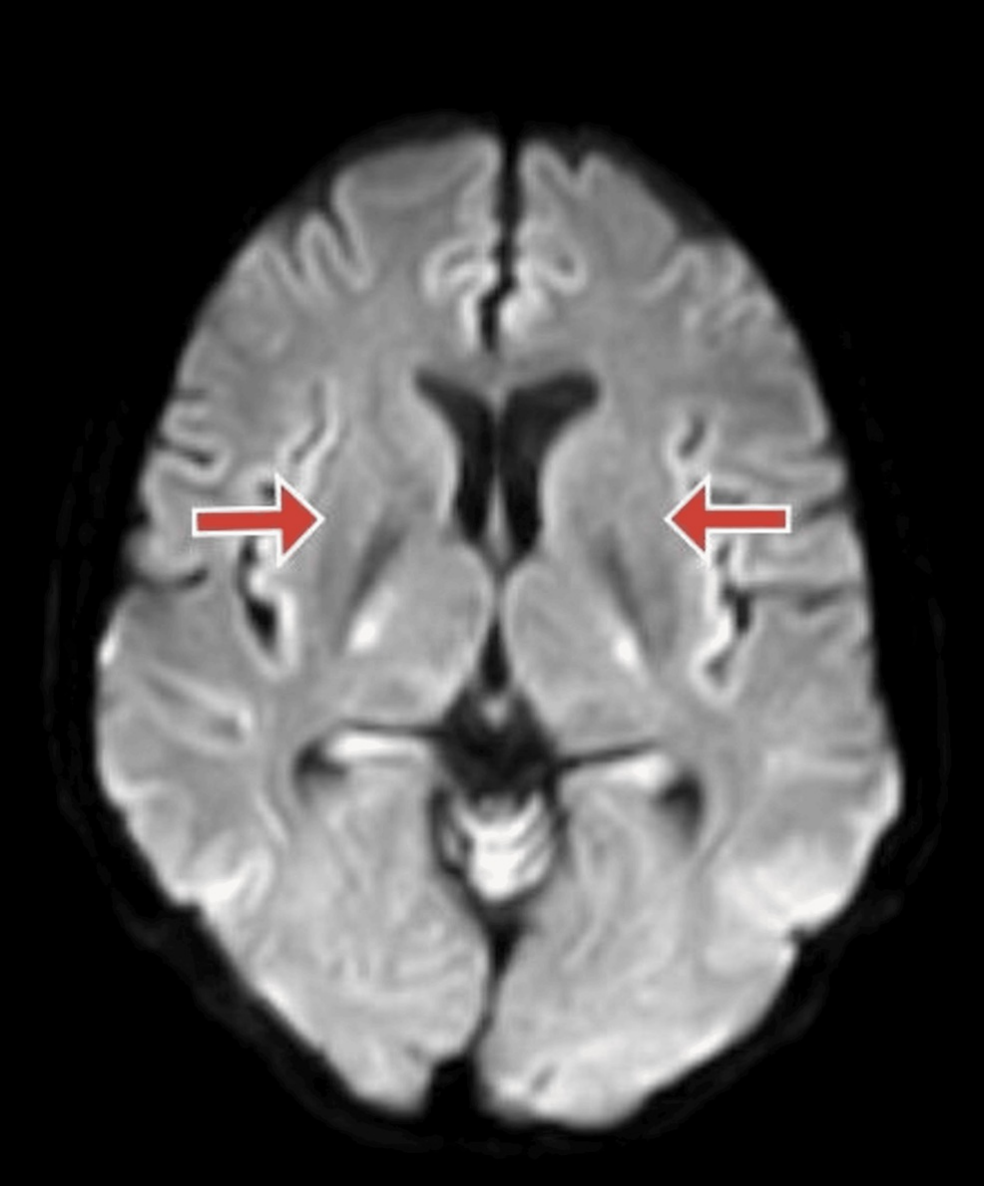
Diffusion-weighted magnetic resonance imaging (DW-MRI) section of the brain DW-MRI section of brain showing restricted diffusion in bilateral basal ganglia.
